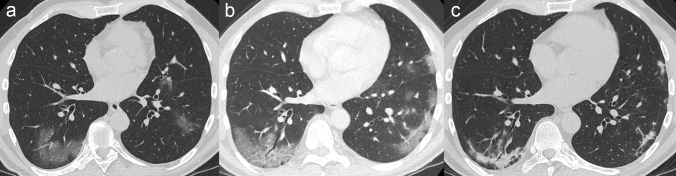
CT scans of a patient with COVID-19 pneumonia demonstrating the typical evolution of the disease by phases. a In the early phase, GG opacities are the predominant finding. b 10 days after the onset of symptoms (progressive to peak phase) more extensive GG areas with crazy paving appearance and typical distribution at the lung periphery are observed. c One week later, GG areas decline and lead to the appearance of multifocal consolidation with mild parenchymal distortion
